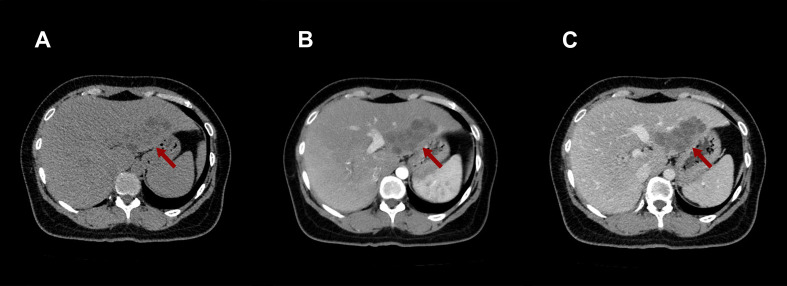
Upper abdominal CT imaging examination. (A) Plain scan showed a low-density mass in the left lateral lobe of the liver with blurred boundary. (B) The arterial phase showed mild septal-like enhancement. (C) Mild enhancement persisted during the venous phase. (The mass is indicated by red arrows in all panels.).
